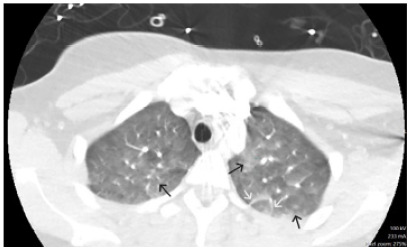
Repeat computed tomography chest post hypoxia. Black arrows indicate ground-glass opacities. White arrows indicate smooth interlobular septal thickening.
